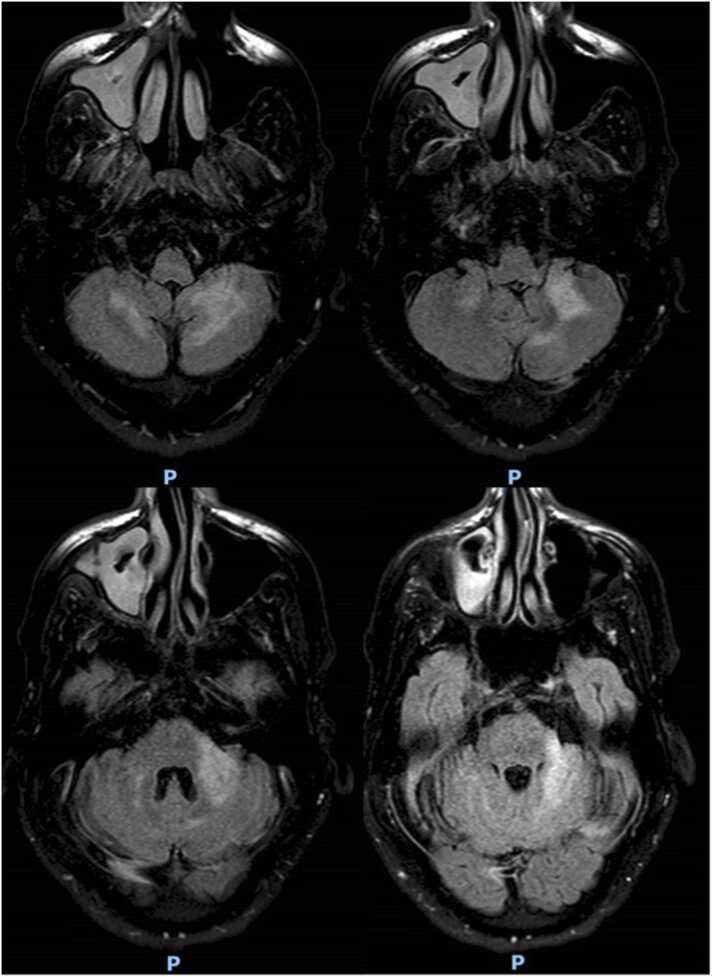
MRI Brain with and without contrast; axial T2 FLAIR (March 23, 2022). Subcortical T2 hyperintense signal abnormality involving the left brachium pontis/left cerebellar hemisphere with possible minimal right cerebellar involvement.
